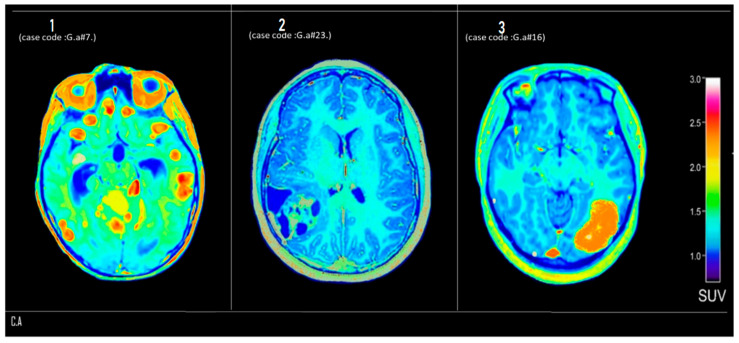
Axial ^18^F-FDG PET brain scans from Center A. Three representative cases demonstrate varying metabolic patterns based on standardized uptake value (SUV) distribution (color bar, right). Internal case codes (G.a #7, G.a #23, G.a #16) refer to anonymized patient identifiers in the study database and are provided for traceability. Panel 1 (G.a #7): diffuse hypermetabolism (SUV > 2.5, red-yellow) in bilateral basal ganglia and orbitofrontal regions, suggestive of inflammatory activity. Panel 2 (G.a #23): global hypometabolism with homogeneous SUV distribution (~1.2–1.6), consistent with a hypometabolic pattern. Panel 3 (G.a #16): focal, well-demarcated hypermetabolic lesion in the right occipitotemporal lobe (SUV ≈ 3.0), suggestive of a neoplastic process.
